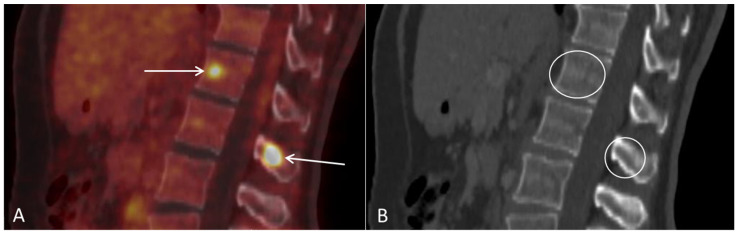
(A) Follow-up sagittal PET/CT fused and (B) sagittal CT after three series of chemotherapy shows metabolic activity in the spine suspicious for bone metastases ((A), arrows), with no corresponding lesions on CT ((B), circles).
